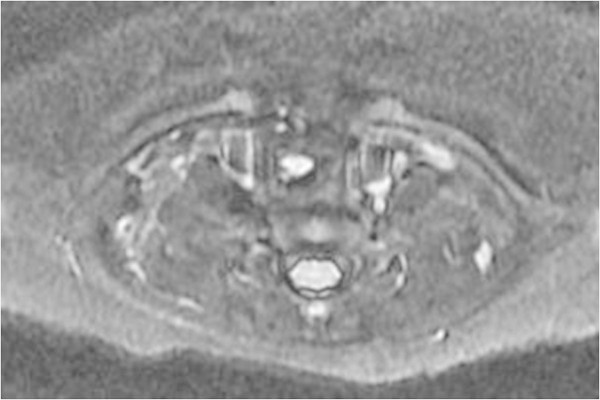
The mass was of high intensity in T2-weighted MRI images.
